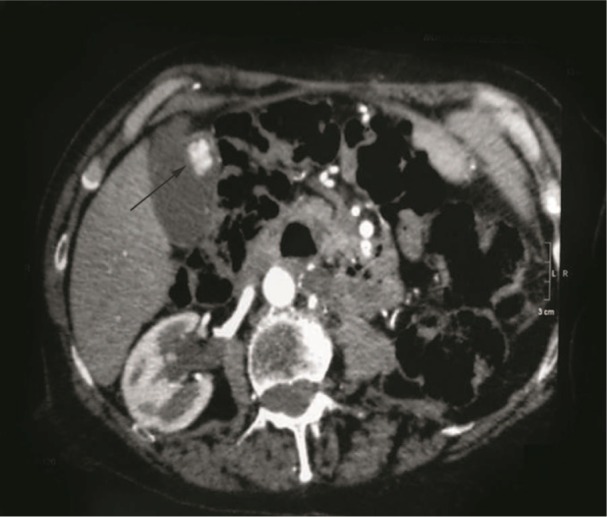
Case 2. Computed tomography image after intravenous contrast enhancement reveals an intraluminal hyperdense enhancing mass (arrow).
